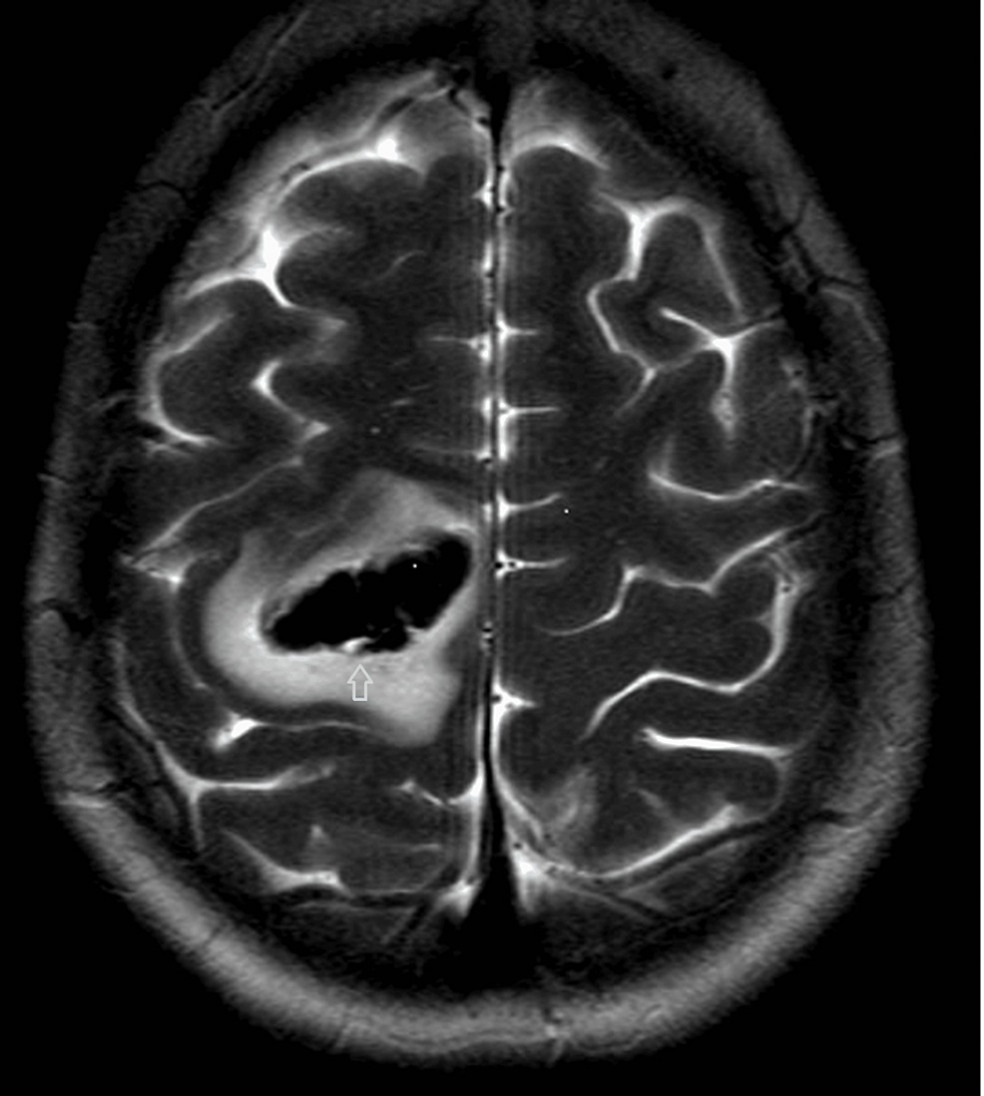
T2 axial MRI image on day 3 shows parenchymal haematoma in right precentral gyrus and posterior aspect of right superior frontal gyrus.
